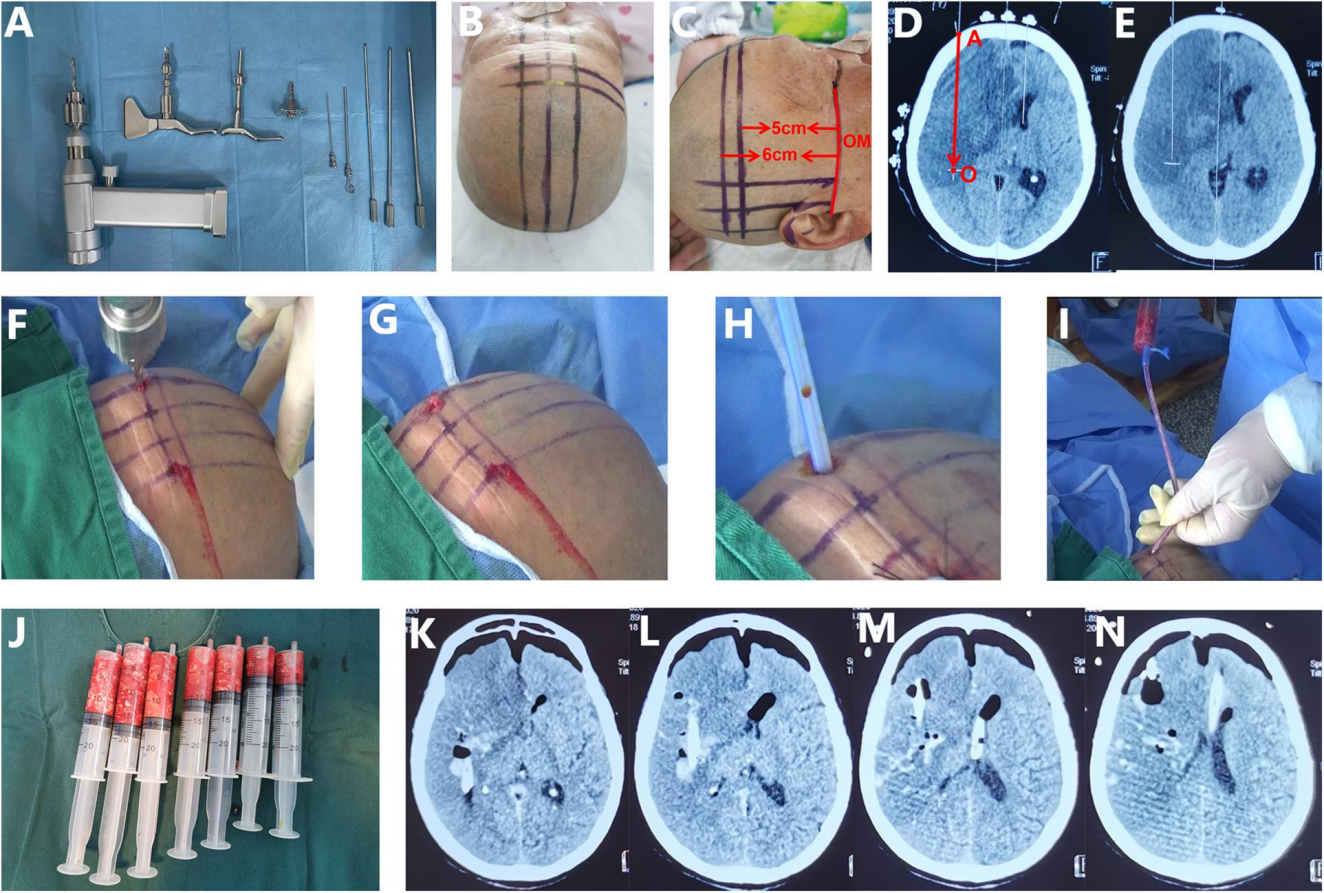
Principle and procedure of cubic stereotactic aspiration of necrotic brain tissue. (A) The main surgical instruments include an electric cranial drill. (B,C) The median sagittal line, the orbitomeatal (OM) line and the horizontal line 5 cm above the OM line were drawn. (D,E) A standard CT slice was obtained through several metal markers fixed on the horizontal line 5 cm above the OM line. The slice showing the largest infarct size was identified at approximately the same level. The puncture pathway “AO” in this case was located on the paramedian sagittal plane, 3.5 cm lateral to the median sagittal line and, on the horizontal plane 5 cm above the OM line. (D–G) A 6 mm bone hole was drilled with an electric cranial drill along a trajectory defined by two mutually perpendicular planes: the horizontal and the parasagittal planes. (H) A 12-French silicone catheter was inserted into the deep part of the infarct lesion. (I) Necrotic brain tissue was aspirated with a 20 mL syringe at a negative pressure of 5 mL from deep to shallow along the puncture pathway. (J) Approximately 70 mL of necrotic tissue was aspirated. (K–N) The immediate postoperative CT scan revealed bilateral collapsed prefrontal tissue, intracranial gas, and reduced midline shift, indicating adequate decompression by aspiration.
